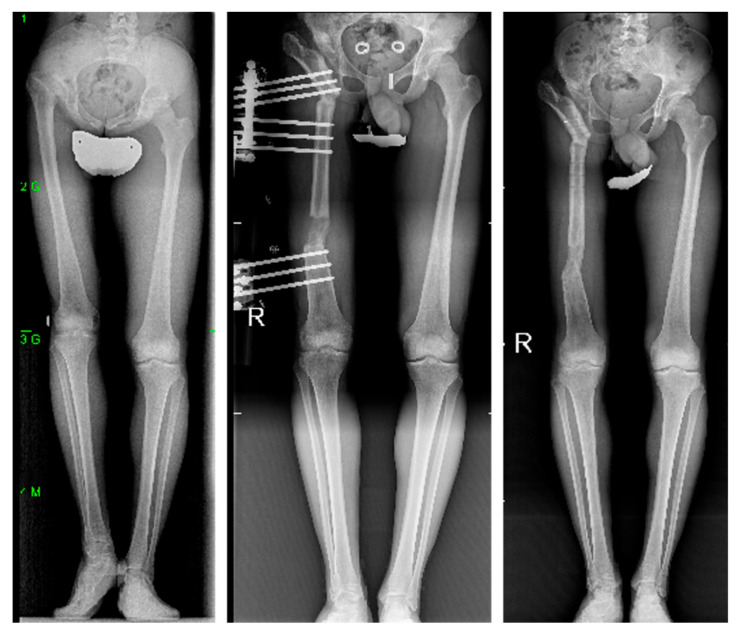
Radiographic evolution of a 15-year-old male with developmental dysplasia of the hip, multiple previous surgeries, a painful stiff hip, a 6 cm leg length discrepancy and severe Trendelenburg gait, treated with an LRS Advanced monolateral external fixator (Orthofix Srl, Verona, Italy).
